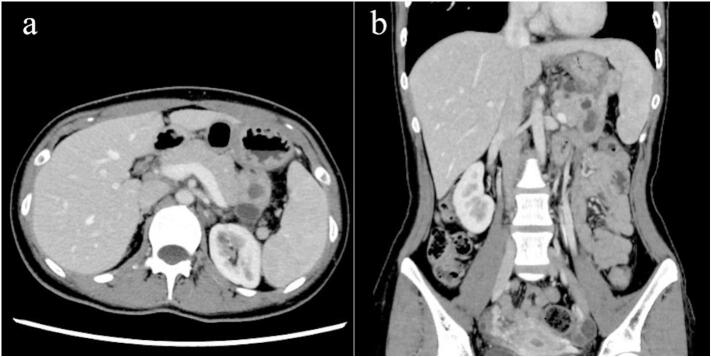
(a) Contrast-enhanced computed tomography (CT) at three months after discharge reveals that the peripancreatic hematoma is remarkably reduced. CT shows a cystic lesion in the tail of the pancreas.
